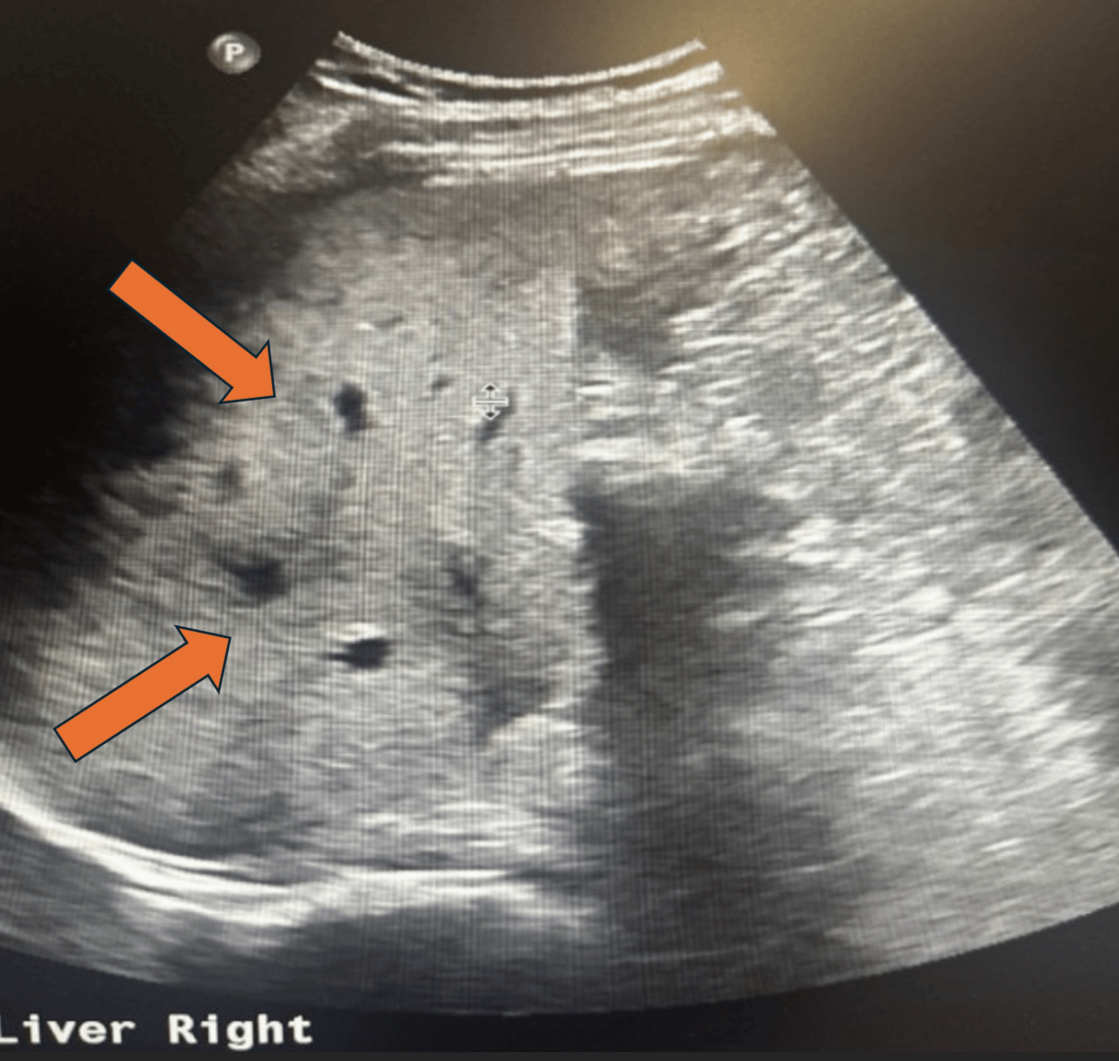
An ultrasound of the liver showing increased echogenicity, suggestive of hepatic steatosis. The orange arrows highlight enhanced liver echogenicity.
